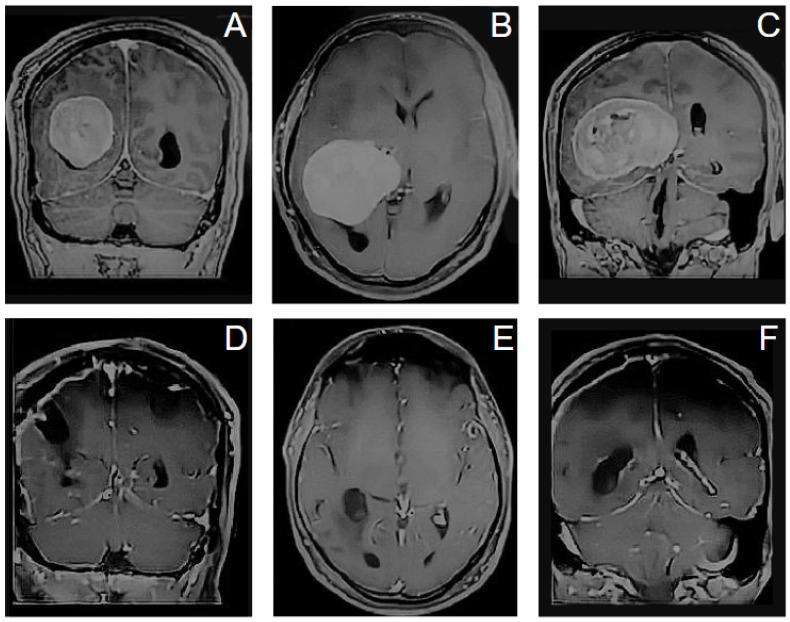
Preoperative (first row (A–C)) and postoperative (second row (D–F)) MRI images showing removal of a large homogeneously enhancing tumor involving the right ventricular trigone that appeared consistent with a meningioma.
